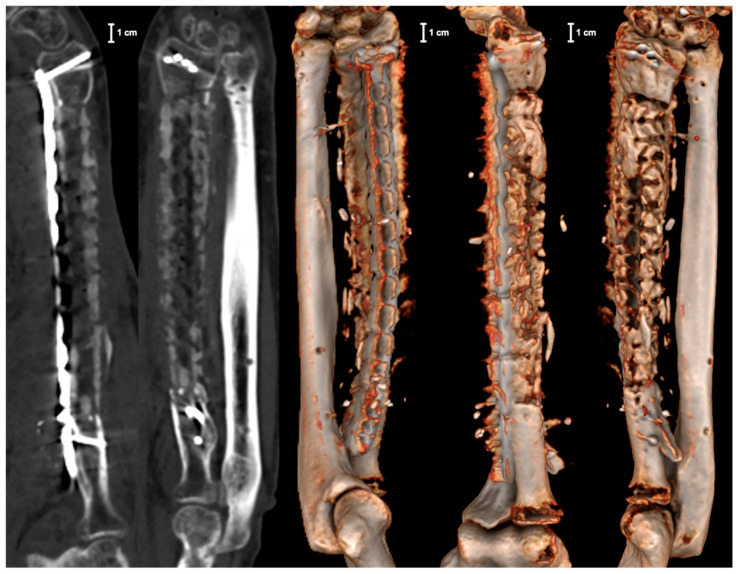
Postoperative computer tomography (CT) of the right forearm.
